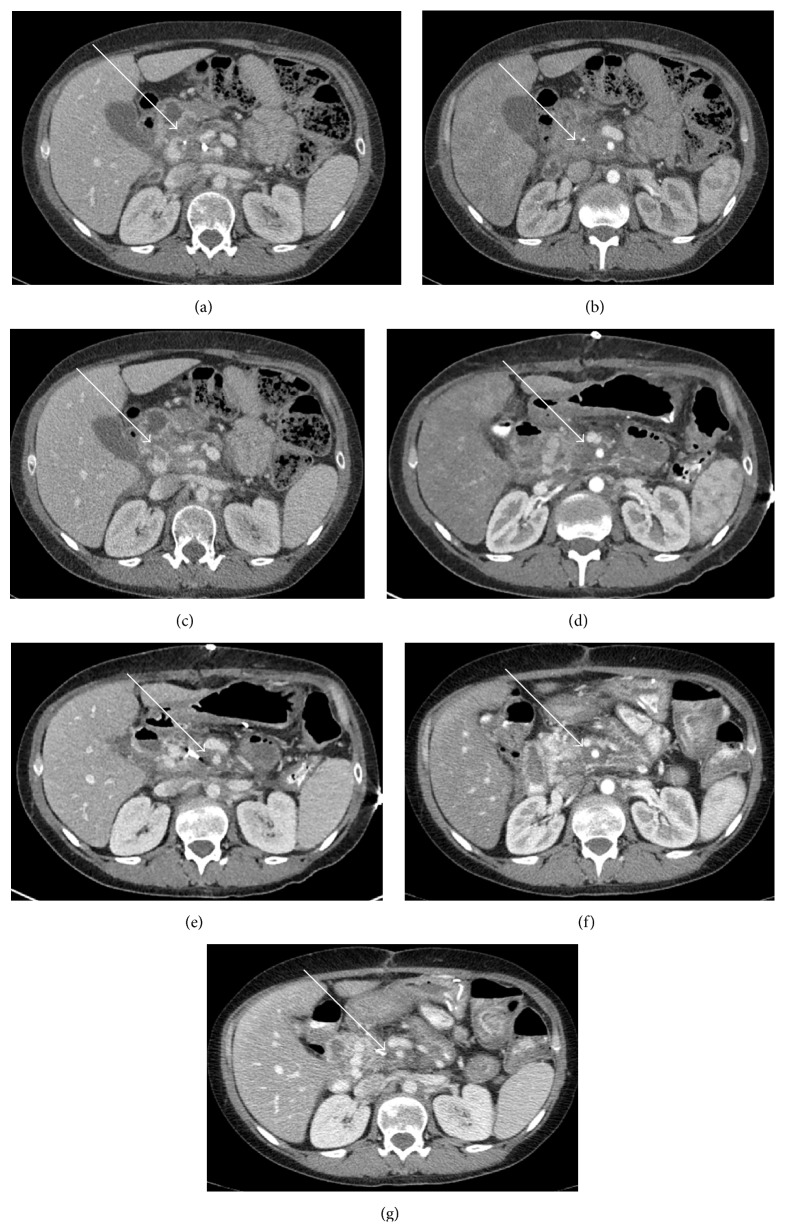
(a) Preoperative CT scan in the portal venous phase displaying abnormal soft tissue in the inferior pancreatic head (white arrow) with a nearby fiducial marker. (b) Arterial phase image displaying soft tissue encasement (white arrow) of superior mesenteric artery (SMA). Another preoperative scan (c) displaying soft tissue encasement along splenic vein and posterior to the portal confluence (white arrow). Immediate postoperative scans in arterial (d) and venous phases (e) displaying an increase in hazy soft tissue in the postablation bed (white arrows) and continued encirclement of SMA. A 2-month postoperative scan in arterial (f) and venous (g) phases displaying similar irregular, hazy, amorphous soft tissue stranding but with decreased size of the postablation zone (white arrow) consistent with no recurrence.
